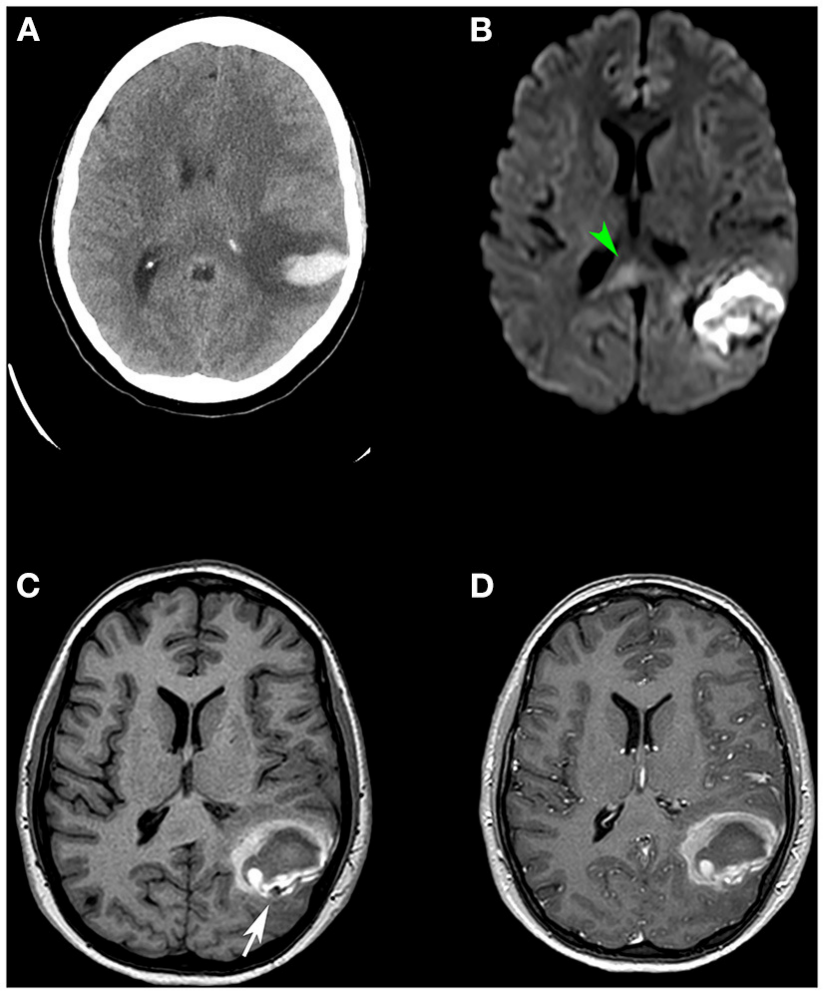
(A) Cranial CT scan showing left temporo-occipital intraparenchymal haematoma. (B) Diffusion-weighted MR image showing increased signal (diffusion restriction) and thickening of the splenium of the corpus callosum (green arrowhead), suggestive of a cytotoxic lesion of the corpus callosum (CLOCC). (C,D) 3D T1-weighted volumetric sequence without contrast (C) and with contrast (D) demonstrating the existence of a nodular image adjacent to the haematoma (white arrow). It presents as a signal void and after contrast administration, it enhances in an analogous way to vascular structures, compatible with a pseudoaneurysmal lesion.
